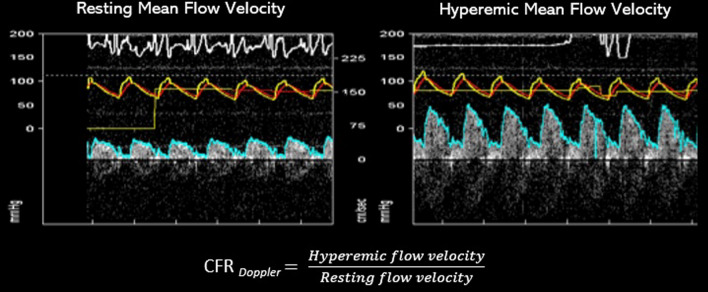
CFR calculation using Doppler. From intracoronary Doppler flow signal, mean coronary flow velocity (CFV) can be derived (blue line depicting the edge of Doppler curves) as the average of several beats. CFR is calculated as the ratio between mean hyperemic CFV and mean resting CFV
